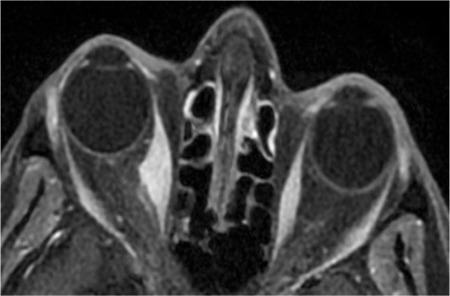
Magnetic resonance imaging of the orbit. Axial postcontrast T1- weighted image showing thickening of the right medial rectus muscle with sparing of the tendon
